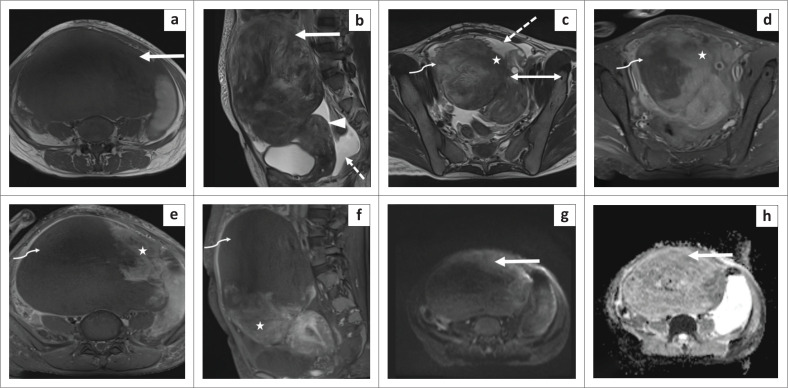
MR imaging in torsion of a subserosal uterine leiomyoma. MR images depict a large solid abdomino-pelvic mass lesion (arrow in a, b) continuous with the uterus (arrowhead in b) in the region of the left cornua (double arrow in c). The mass appears hypointense on the axial T1-weighted image (a) and heterogeneous on sagittal (b) and axial (c) T2-weighted MR images compared to the uterine myometrium. The majority of the mass appears heterogeneously hyperintense on the T2-weighted image (curved arrow in c) with a thin enhancing rim and non-enhancing core on post-contrast axial (d, e) and sagittal (f) MR images (curved arrow). A small juxtauterine area of relatively homogeneous T2 isointense signal (⋆ in c) and post-contrast enhancement (⋆ in d–f) is seen adjacent to its site of uterine attachment. The mass showed no diffusion restriction on diffusion weighted imaging (g) and the corresponding apparent diffusion coefficient map (h).
